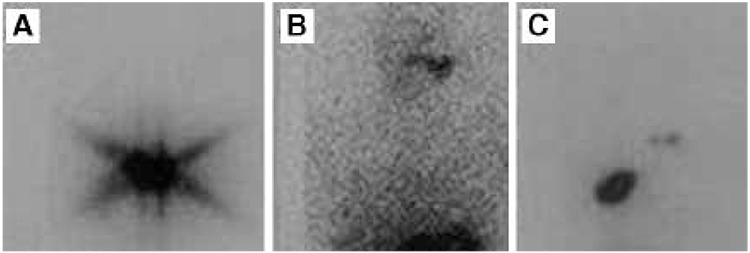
Shows post-therapeutic and follow up I-131 whole-body scintigraphy images of a 55-year old male patient operated for follicular thyroid cancer and received 100 mCi radioactive iodine treatment. A) I-131 whole-body scintigraphy performed 10 days after radioactive iodine therapy displayed high activity in the cervical region by residual tissue, B) I-131 whole-body scintigraphy of the same patient performed at 6 months after radioactive iodine therapy showed no activity consistent with ablation of residual tissue, C) I-131 whole-body scintigraphy of the same patient performed about 12 years after radioactive iodine treatment revealed increased focal activity in the thyroid bed consistent with recurrent malignancy, as well as intense uptake in the right upper thoracic region as a result of newly developed bone metastasis to the scapula (stimulated serum thyroglobulin level of the patient during this period was 187 ng/dl)
